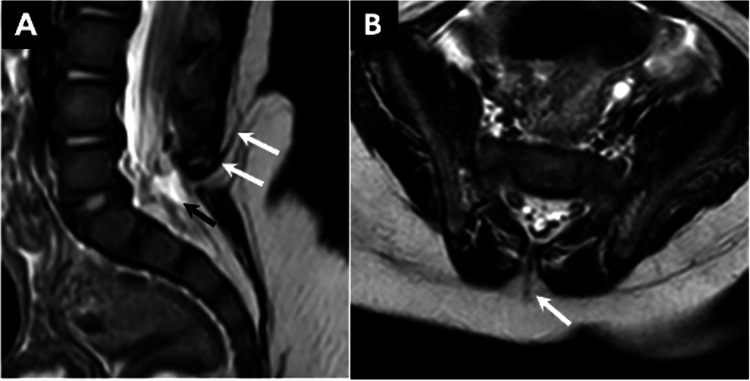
DST. Coronal T2W image (A) demonstrates a curvilinear hypointense tract (arrows) extending from the subcutaneous tissue into the spinal canal at the L5-S1 level, consistent with a DST. An 8 mm concomitant intradural lipoma at the L5 level (black arrow) and tethering of the lower cord are noted. The axial T2-weighted image (B) reveals a posterior midline osseous defect at the L5 level, with a tract connecting the posterior subcutaneous fat to the epidural fat. (white arrow). DST, dermal sinus tract All images were obtained by the authors from the institutional picture archiving and communication system (PACS). They have been de-identified and used in compliance with institutional policies.
